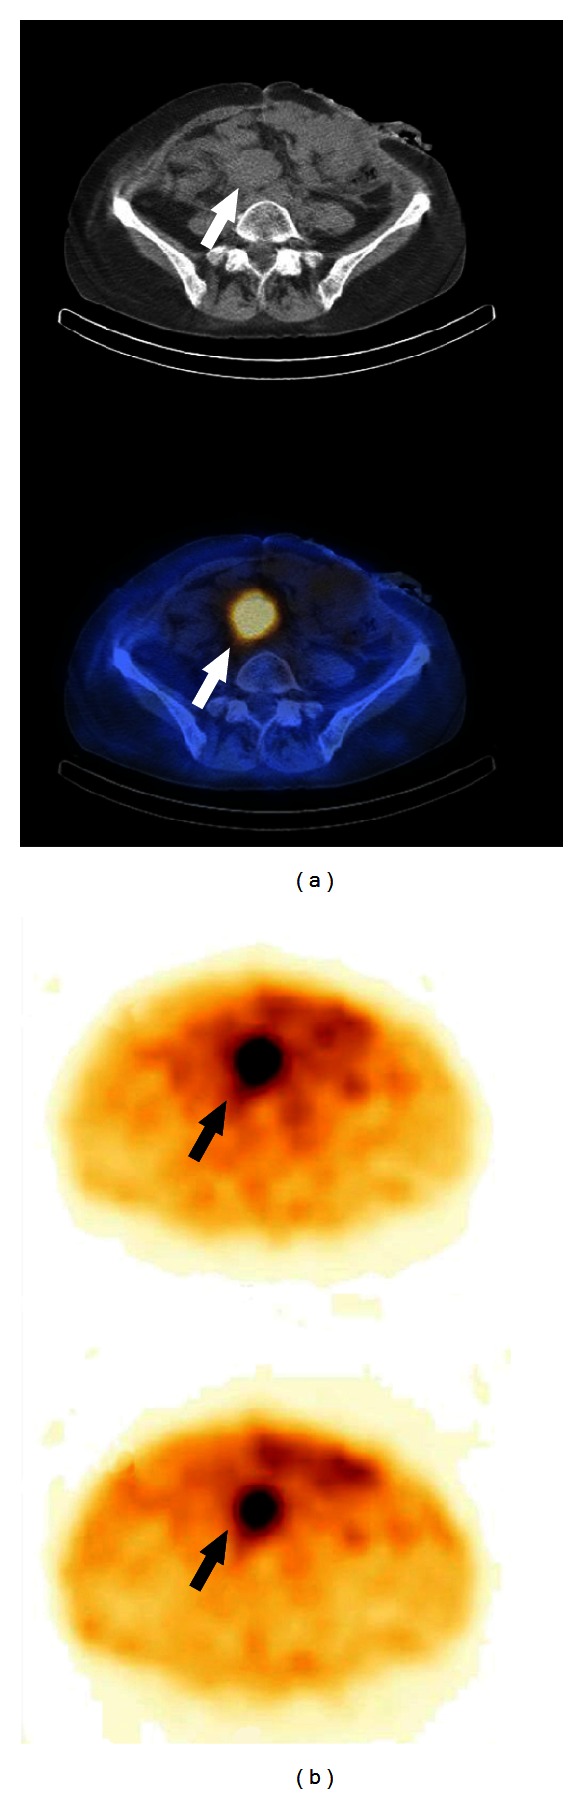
56-year-old woman after ileum resection for carcinoid 15 months ago. Somatostatin receptor imaging with ^111^In-pentetreotide, abdomen CT, SPECT and SPECT/CT, transversal slices. Metastasis of carcinoid in the preaortal lymph node (*arrow head*).
